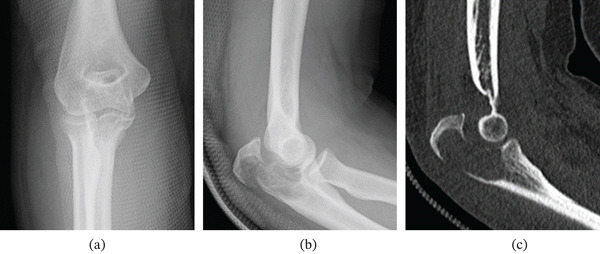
Preoperative plain radiographs and computed tomography (CT) demonstrating an osteolytic lesion of the olecranon with markedly displaced pathological fracture involving the articular surface. The size and thickness of the proximal fragment are preserved, and the normal curvature of the articular surface is disrupted. (a) Anteroposterior radiograph. (b) Lateral radiograph. (c) Sagittal CT image.
